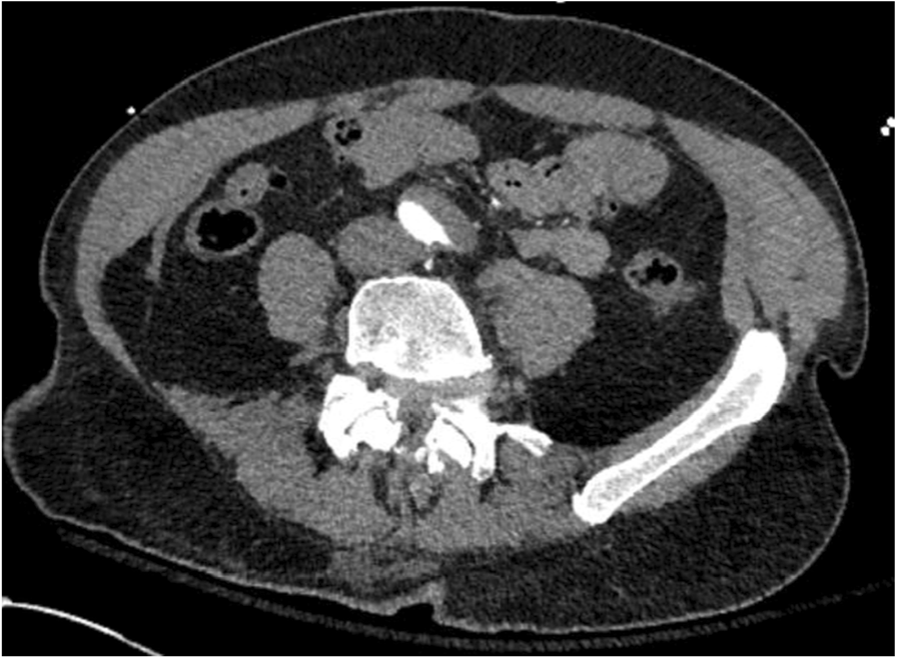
CT angiography demonstrating continued dissection throughout the abdominal aorta to involve the left common iliac artery and bilateral external iliac arteries
